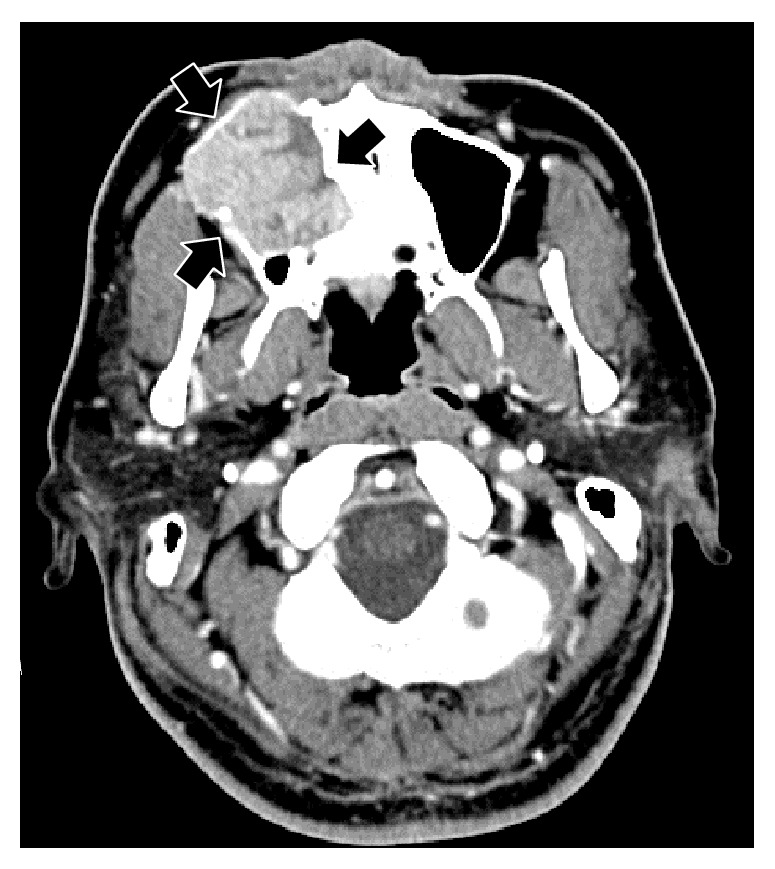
An axial CT image shows a soft tissue density mass eroding the anterior and lateral walls of right maxillary sinus (arrows).
